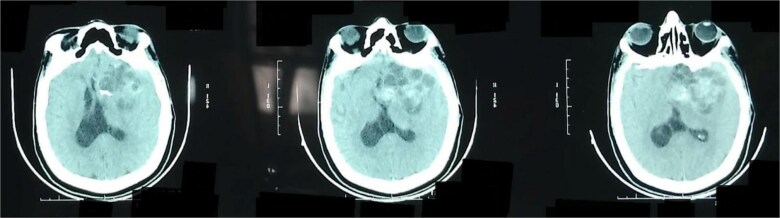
CT scan revealing a recurrent lesion in the right frontotemporal lobe, extending to the suprasellar region. The space-occupying lesion exerts a mass effect on both the lateral and third ventricles, contributing to ventricular compression.
